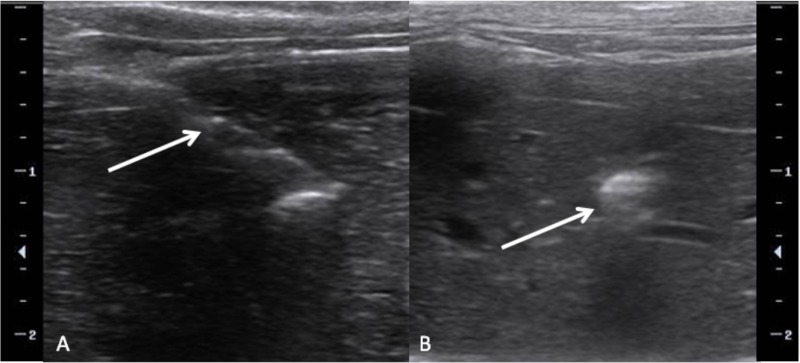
Ultrasound guided implantation. (A) Using ultrasound guidance, a 17 gauge coaxial introducer (arrow) is advanced into the liver. The sharp inner stylet is removed and a small tumor fragment (3-4mm^3^) is placed in the hub of the introducer and pushed with the blunt stylet. (B) Post implantation US image reveals a small focus of hyperechogenicity (arrow) representing the tumor fragment and air.
